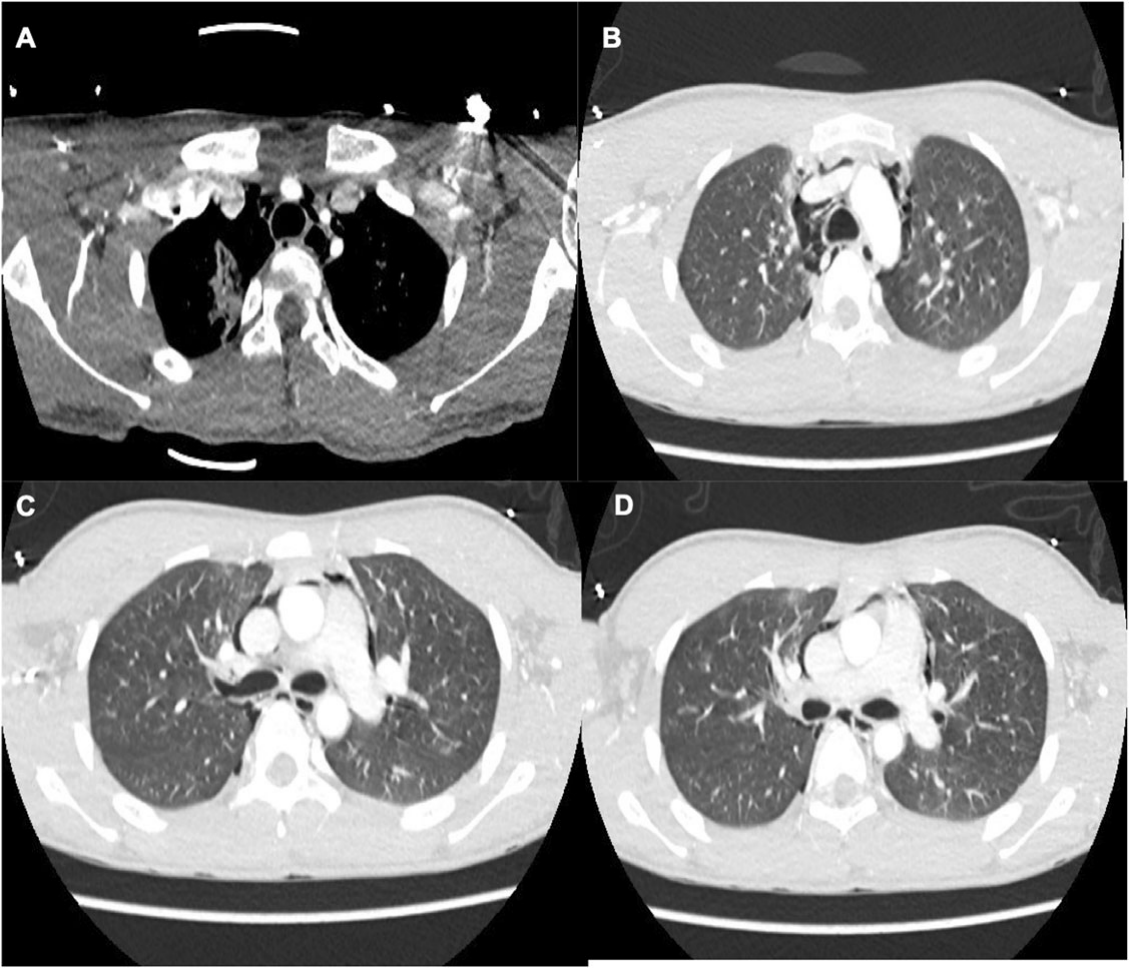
CT angiography. a Pulmonary contusion in the apical portion of the right lung and mediastinal emphysema. b, c, d Mediastinal emphysema dissecting central structures
